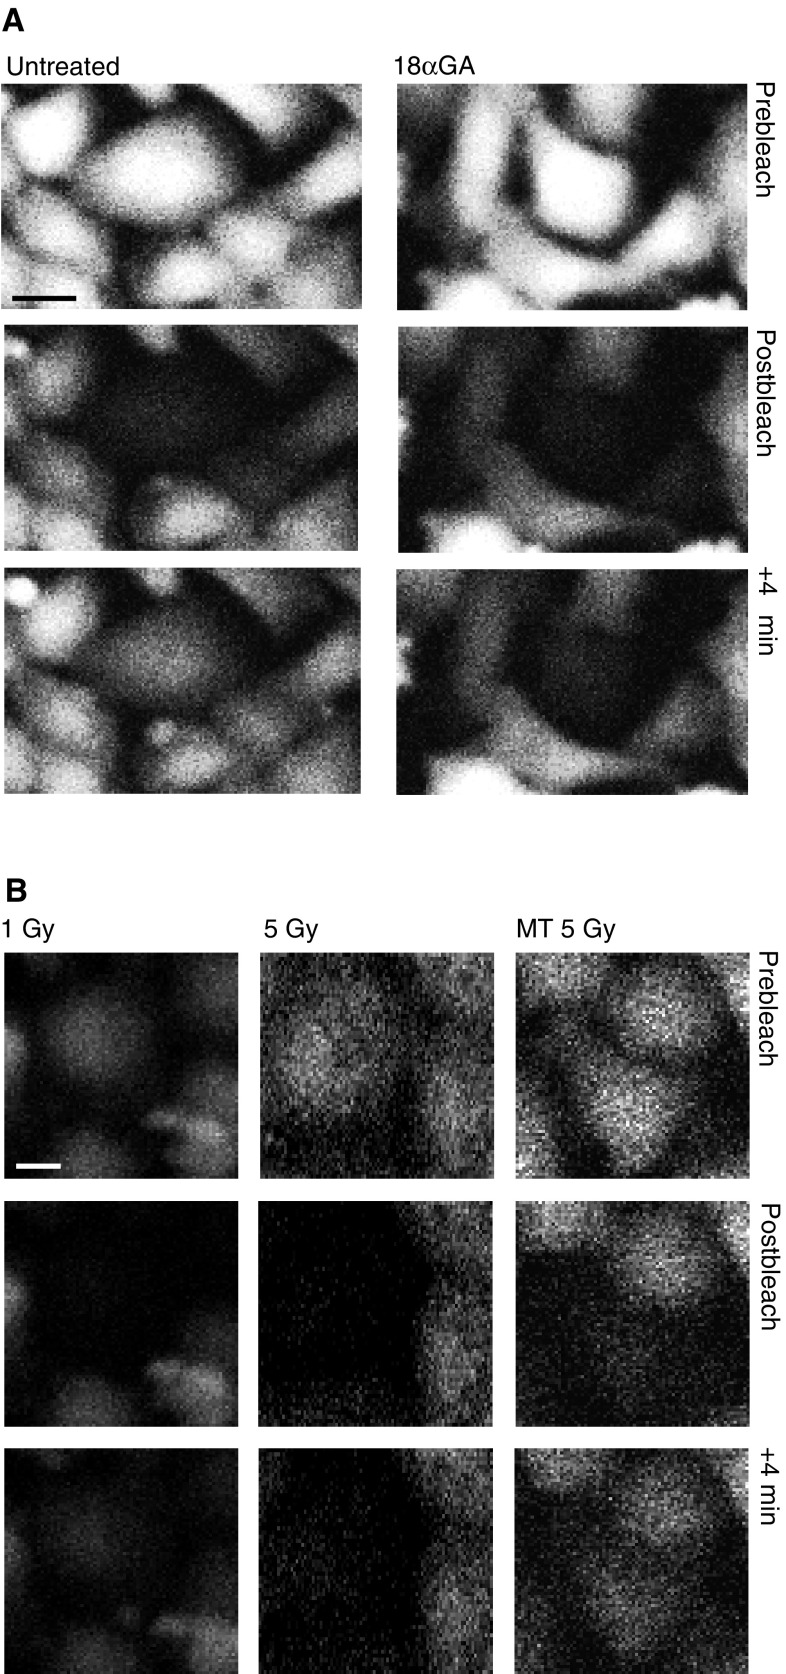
Confocal fluorescence images of WB-F344 cells demonstrate the GapFRAP assay. Prebleach, postbleach and +4 min postbleach are shown in (A) untreated cells and cells treated with 25 *μ*M 18*α-*glycyrrhetinic acid (18*α*GA; gap junction inhibitor) and (B) cells directly exposed to 1 Gy ultrasoft X-rays (1 Gy), cells directly exposed to 5 Gy ultrasoft X-rays (5 Gy) and unirradiated cells treated for 15 min with media transferred after 15 min from cells that had been exposed to 5 Gy ultrasoft X-rays (MT 5 Gy). Bar represents 10 *μ*m.
